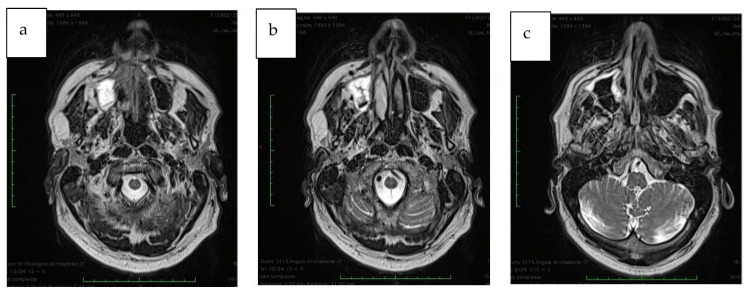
(a–c) MRI section showing the extension of the maxillary lesion.
